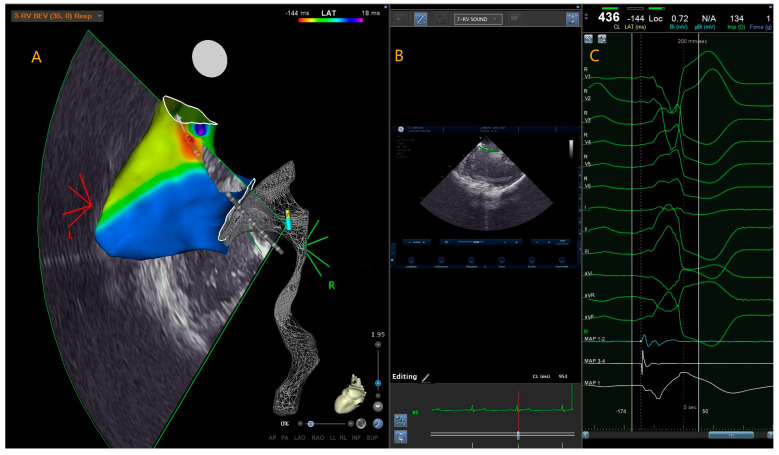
This is a local activation time map of a 36-year-old leisure-time athlete with idiopathic premature ventricular contractions originating from the right ventricular outflow tract. The colorimetric map indicates that the primary site of onset of premature ventricular contractions is the posterior right ventricular outflow tract. In this region, the ablator catheter is visible in transparency, with the tip at the level of the region of interest (A). Intracardiac echocardiography and the CARTOSOUND^®^ Module with SOUND-STAR^®^ can be used to integrate the electro-anatomical map with the intracardiac echocardiographic map. This allows for visualisation of the catheter and its tip position using ICE (B). The bipolar ablator catheter signal is more advanced than the surface QRS signal, and the unipolar signal is represented by a QS signal (C). PVC (premature ventricular complex); RVOT (right ventricular outflow tract); ICE (intra cardiac echocardiography).
